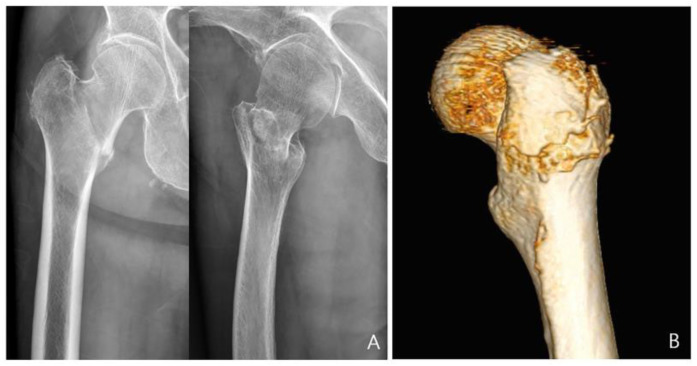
Radiographic images of a patient with a basicervical femoral fracture. In the anteroposterior view, the fracture appears as a two-part fracture, and the comminution of the greater trochanter is not distinctly visible in the lateral view (A). A minimally displaced fracture of the greater trochanter is evident on the 3D CT image (B).
